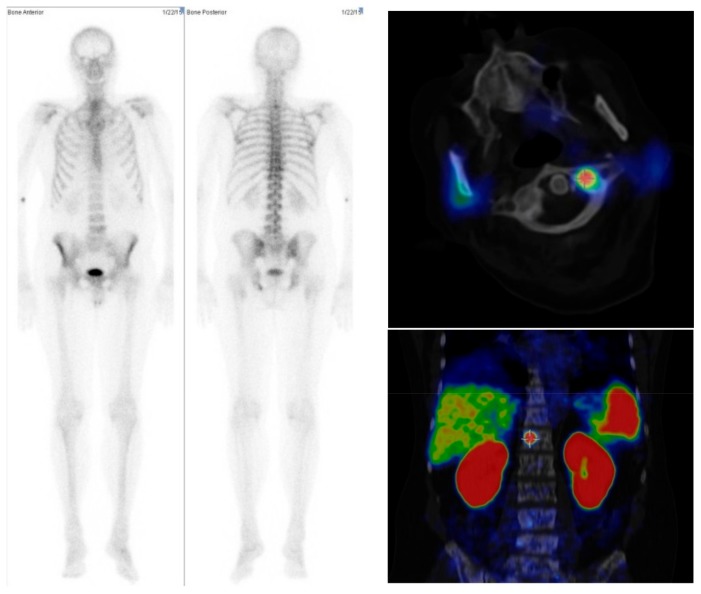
Example of a case in which the semiautomatic tool showed no lesions suspicious for metastases in the bone scan images (Left and Middle), while the visual analysis suspected bone lesions in the cervical, thoracic, and lumbar spine. PSMA-PET/CT agreed with these findings (Right).
